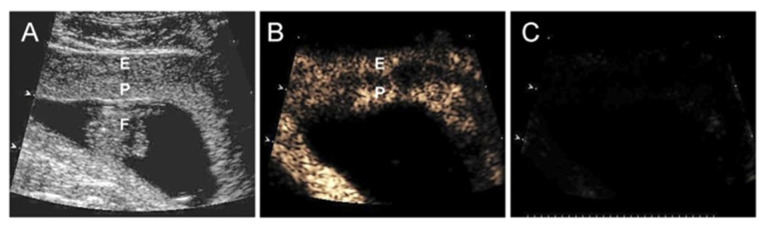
(A) Grayscale Doppler image of the endometrium (E), placenta (P), and fetus (F), and CEU images (B) before and (C) after the collapse of the MBs/uncoupling of VEGF DNA on day 45 of gestation in an E_2_-treated baboon. Reproduced with permission from [82].
